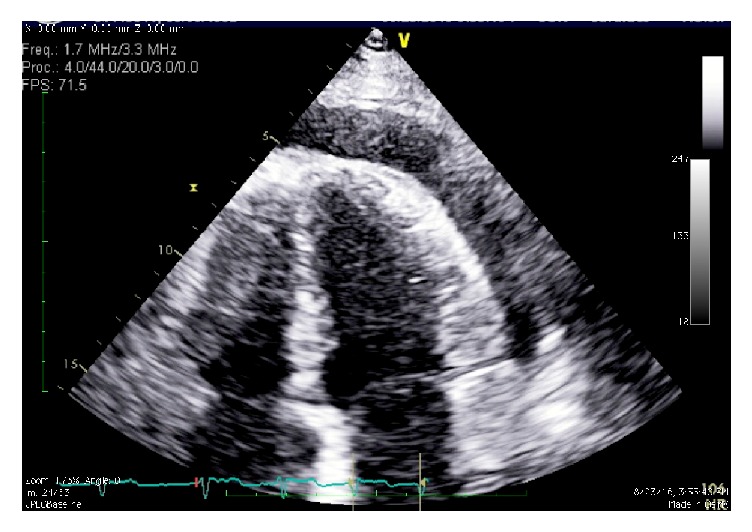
Two-dimensional transthoracic echocardiogram showing a large pericardial effusion in the apical view.
